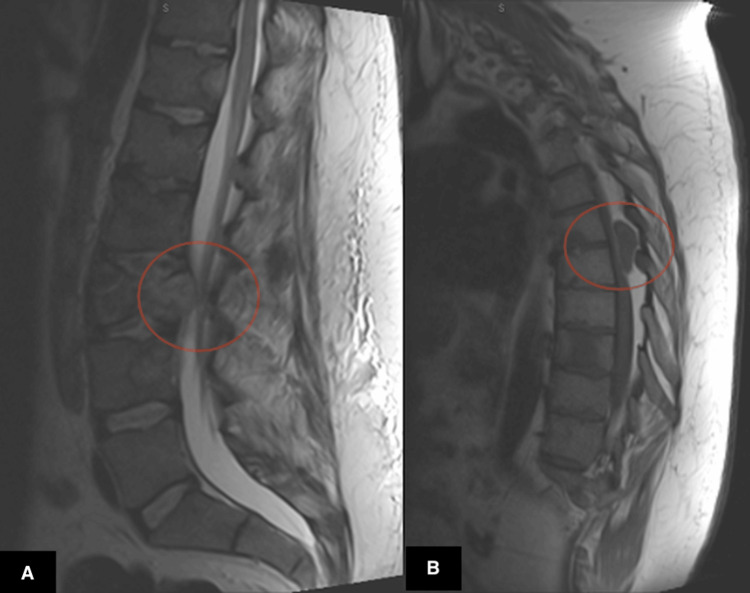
Sagittal T2-weighted MRI of the thoracic and lumbar spine obtained in November 2025 demonstrating epidural metastatic disease with spinal canal stenosis Magnetic resonance imaging of the thoracic and lumbar spine, demonstrating an epidural tumor with spinal canal compromise. (A) Sagittal lumbar spine image demonstrating a pathologic compression fracture of the L3 vertebral body with associated expansile soft tissue mass extending into the epidural space and impinging upon the thecal sac, producing moderate to severe spinal canal stenosis. (B) Sagittal thoracic spine image demonstrating an enhancing extradural mass in the posterior spinal canal at the T6-T7 level measuring approximately 3.4 × 1.1 × 1.1 cm, resulting in epidural spinal canal narrowing consistent with metastatic epidural disease MRI: magnetic resonance imaging
